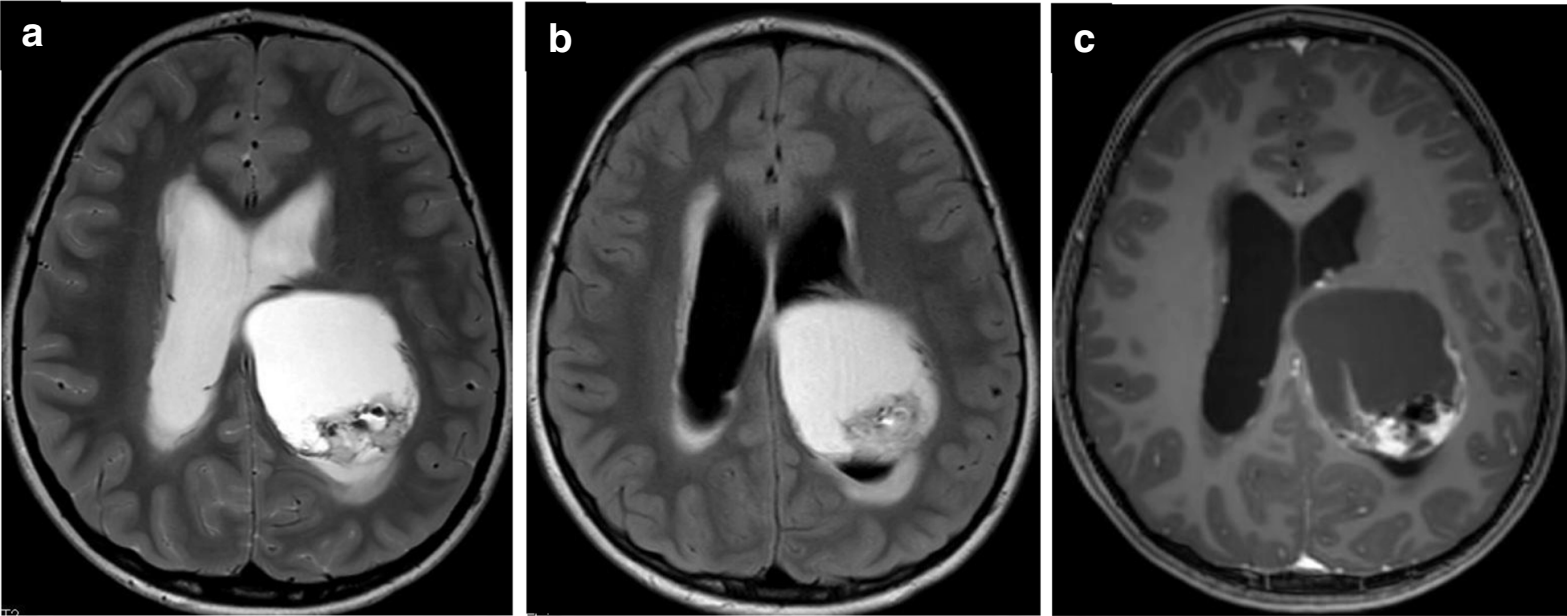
Pilocytic astrocytoma with *BRAF-KIAA* 1549 fusion in a 10-year-old male presenting with seizure. Axial T2 (a), FLAIR (b), and T1-weighted contrast-enhanced (c) MR images show a predominantly cystic, T2/FLAIR-hyperintense left frontoparietal periventricular tumor with a solid, avidly-enhancing mural nodule
